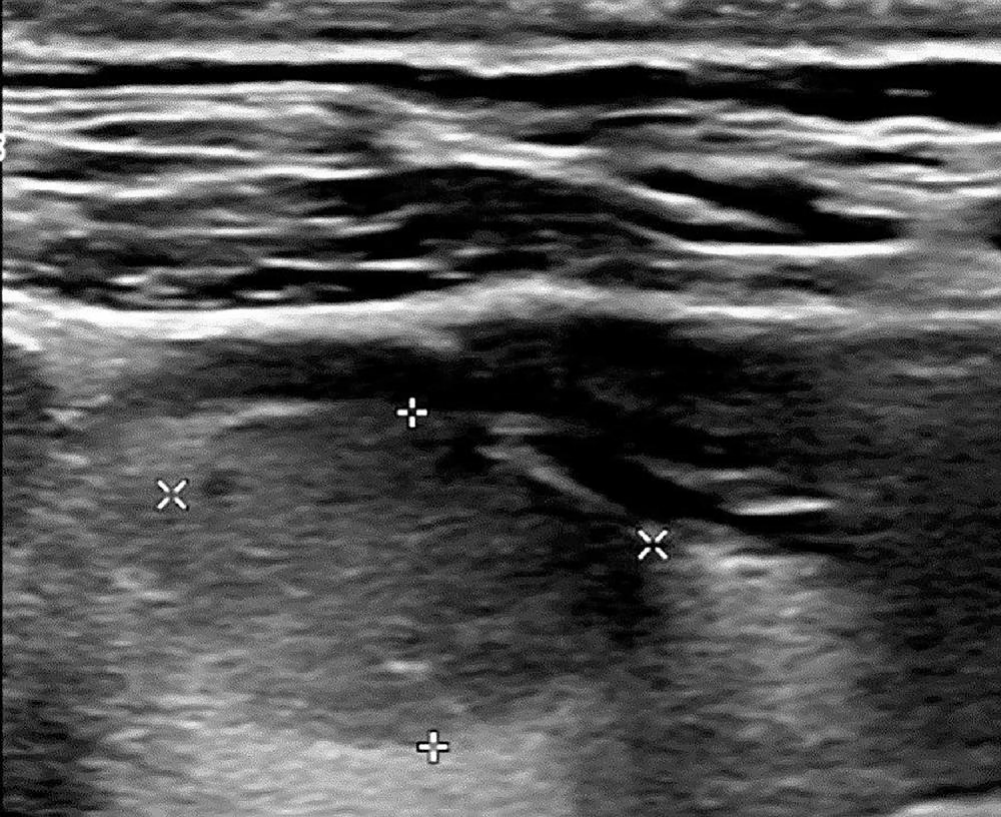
Neck ultrasound reveals a 1.1 × 1.5 × 1.9 cm nodule in the right lobe with the following characteristics based on the ACR TI-RADS: solid composition (2 points), hypoechoic echogenicity (2 points), wider-than-tall shape (0 points), smooth margin (0 points), and no echogenic foci (0 points). The total score is 4 points, classifying the nodule as TI-RADS 4. Abbreviations: ACR-TI-RADS, American College of Radiology Thyroid Imaging Reporting and Data System.
